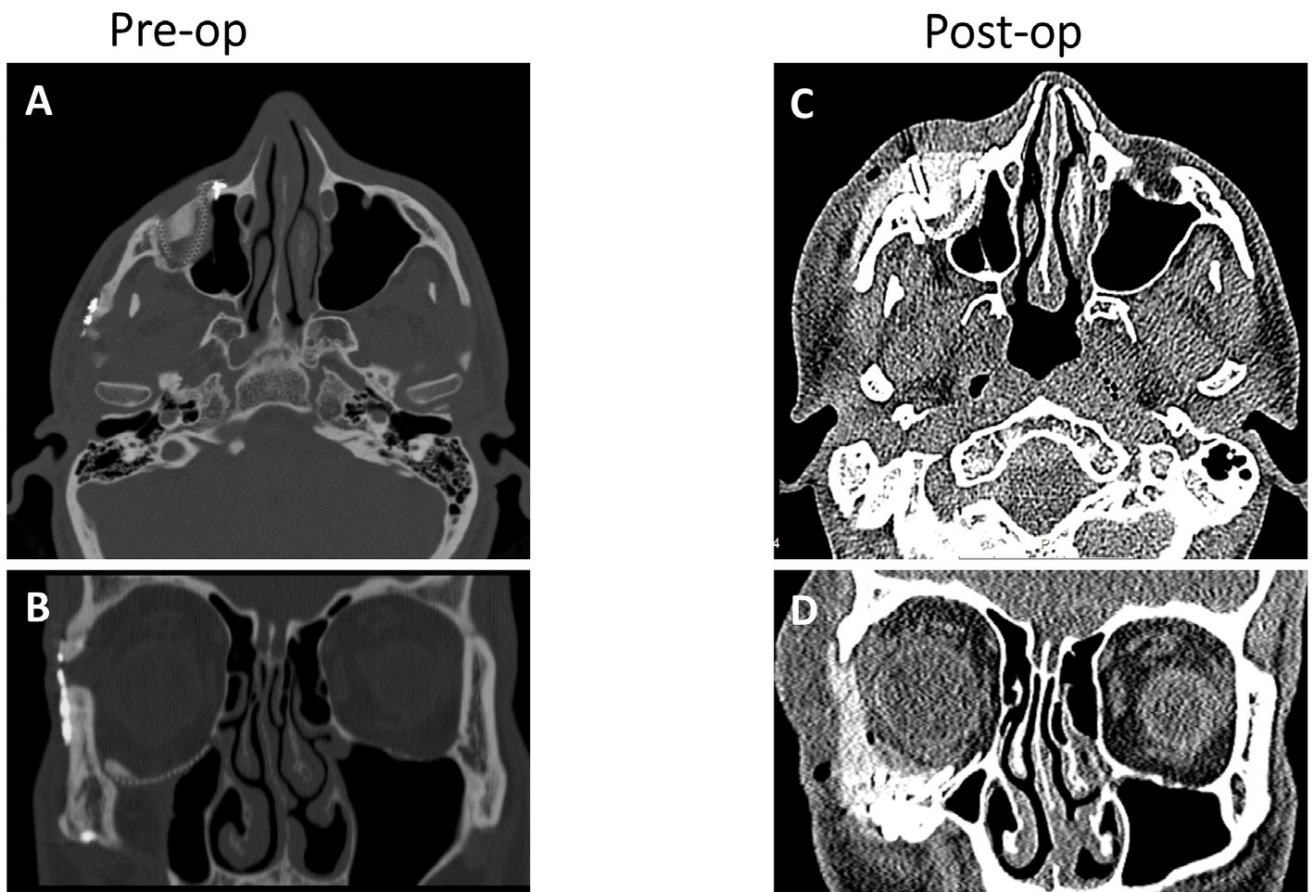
Pre- and Postoperative Computed Tomography Imaging of the patient in Figure 11. A and B: Axial and coronal computed tomography reconstructions, respectively, showing the deficiency in the right malar eminence and the increased orbital volume. C and D: Axial and coronal computed tomography reconstructions, respectively, showing proper orbital volume and projection of the malar eminence. Notice the need for soft tissue window computed tomography reconstruction as the polyether ether ketone (PEEK) implant does not have the same radiopacity as bone.
